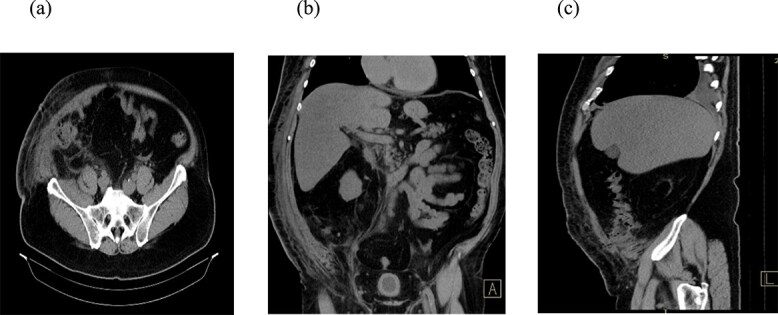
CT scan of the abdomen. Three reconstructions were evaluated: Axial (a), coronal (b), and sagittal (c). The findings suggest a collision of the cecum with the anterior abdominal wall at the site of the previous laparotomy incision, accompanied by surrounding mesenteric stranding. In the region of the anterior abdominal wall, there is fat stranding within the subcutaneous tissue, indicating inflammation, as well as air inclusions originating from the cecum, findings consistent with a colo-cutaneous fistula.
